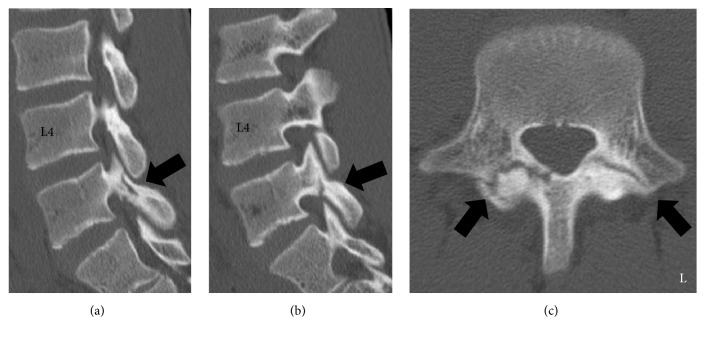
Reconstructed computed tomography (CT) scan of the lumbar spine from the first visit to a previous doctor's office. (a) Right parasagittal reconstructed CT image. (b) Left parasagittal reconstructed CT image. (c) Axial CT image at L5 level. Fissures (black arrows) in the bilateral pars interarticularis of the L5 can be seen (a, b, c). These radiological findings indicated a bilateral spondylolysis of the L5.
